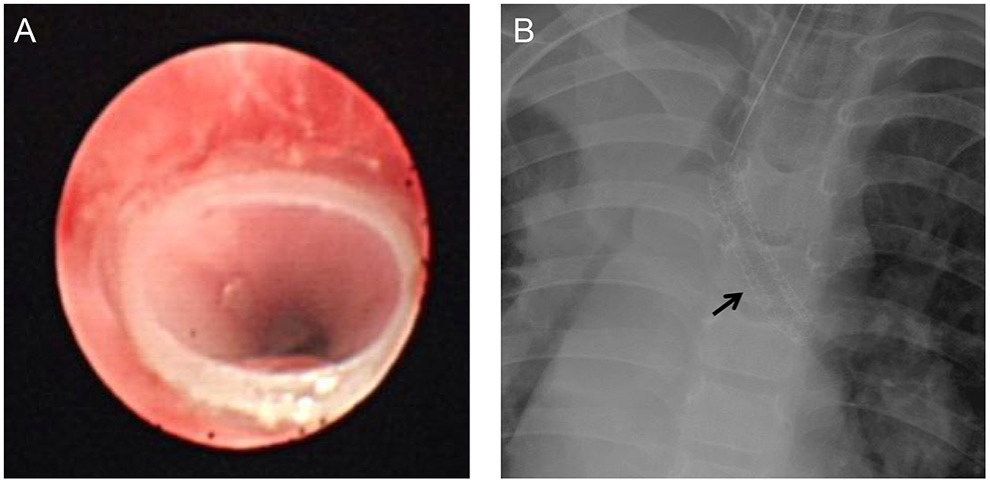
Post-operative image of fiberoptic bronchoscopy stent implantation (A) and chest X-ray [(B), arrow].
